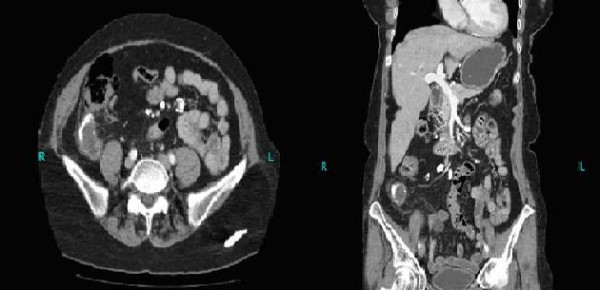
Computed tomography scan showing calcified appendiceal mucocele and calcified mesenteric lymph nodes.
